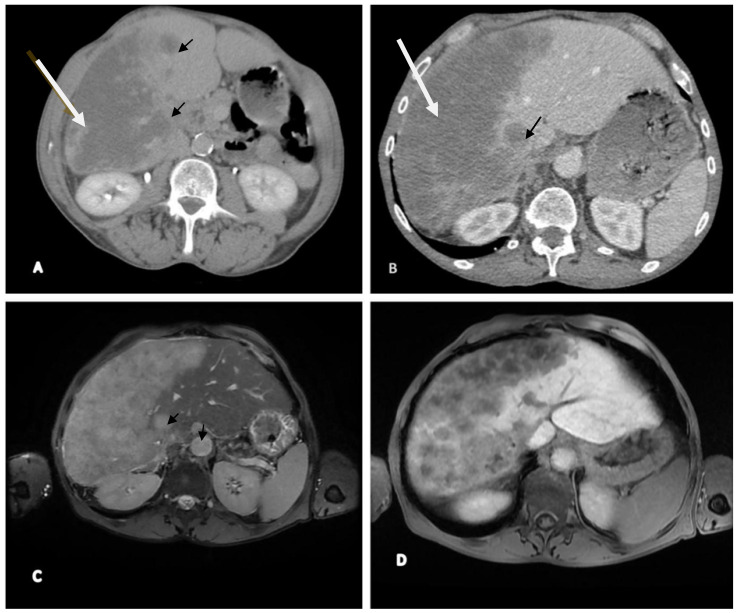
CT (A,B) and MRI (C,D) examination. Contrast-enhanced axial CT images of the liver acquired at multiple phases demonstrate a large, well-defined enhancing mass located in the right hepatic lobe (indicated by the large arrow), consistent with primary hepatocellular carcinoma (HCC). Additionally, a smaller enhancing lesion is observed in the left hepatic lobe (indicated by the small arrow), suggestive of intrahepatic metastasis. (A) On the non-contrast (plain) scan, both lesions present as hypodense relative to the surrounding liver parenchyma. (B) During the arterial phase, both lesions exhibit early arterial enhancement characteristic of HCC. Liver enlarged, heterogeneous structure, irregular contour, lobulated at the level of the left lobe, almost entirely occupied by multiple nodular lesions, both isolated and well-defined, with axial diameters up to approximately 3.3 cm (C). In T2 hyperintensity and T1 hypointensity, with moderate diffusion restriction in DWI/ADC sequences, post-contrast enhancement shows hypointense lesions with multiple non-enhancing areas inside (most likely necrosis), and areas that compress the right portal branches, which appear filiform and homogeneously opacified post-contrast. Lesions exhibit hypointensity compared to the surrounding parenchyma, with characteristics of malignancy—HCC with secondary hepatic lesions and associated perfusion disturbances (D).
